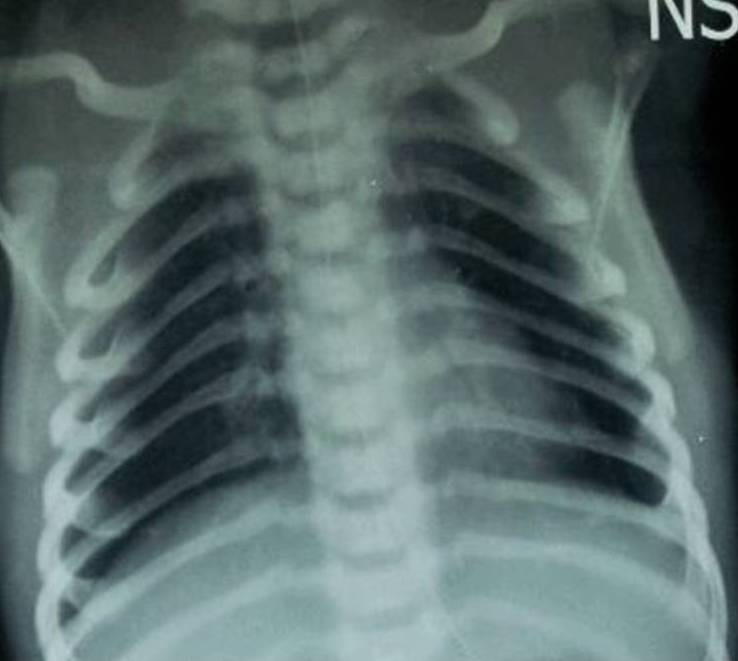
Figure 2:Chest X-ray (AP view) showing resolution of bilateral pneumothoraces
